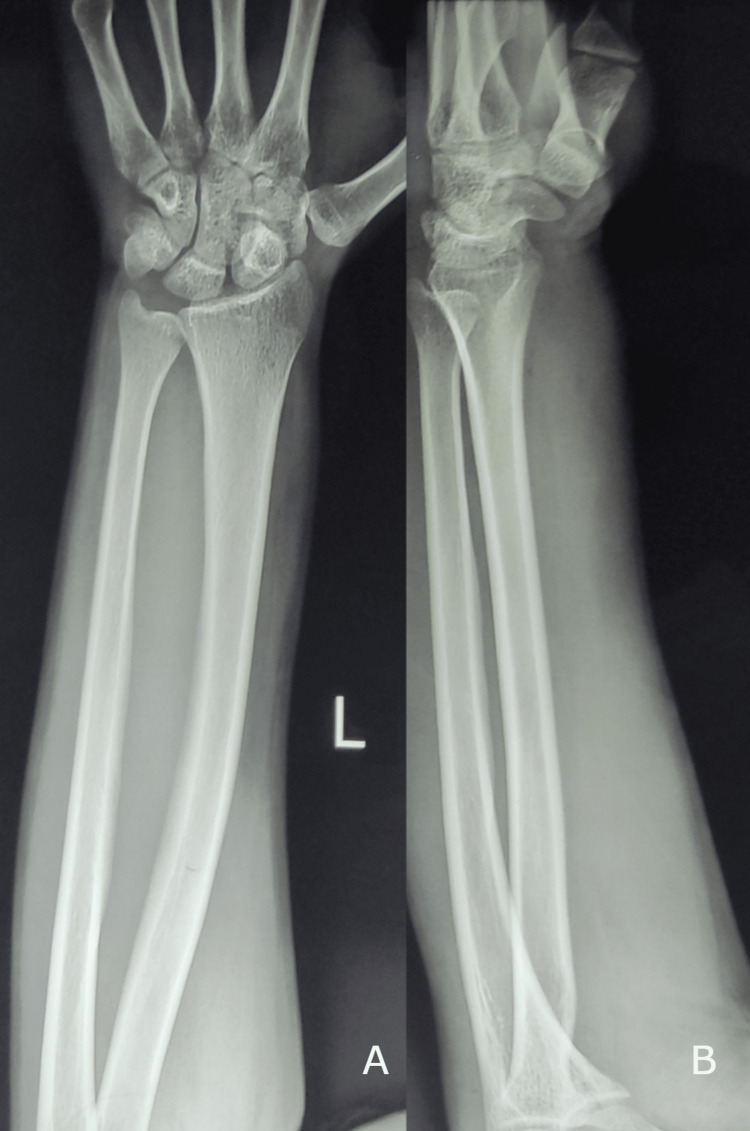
Radiographs of the forearm and wrist revealed no significant abnormality
